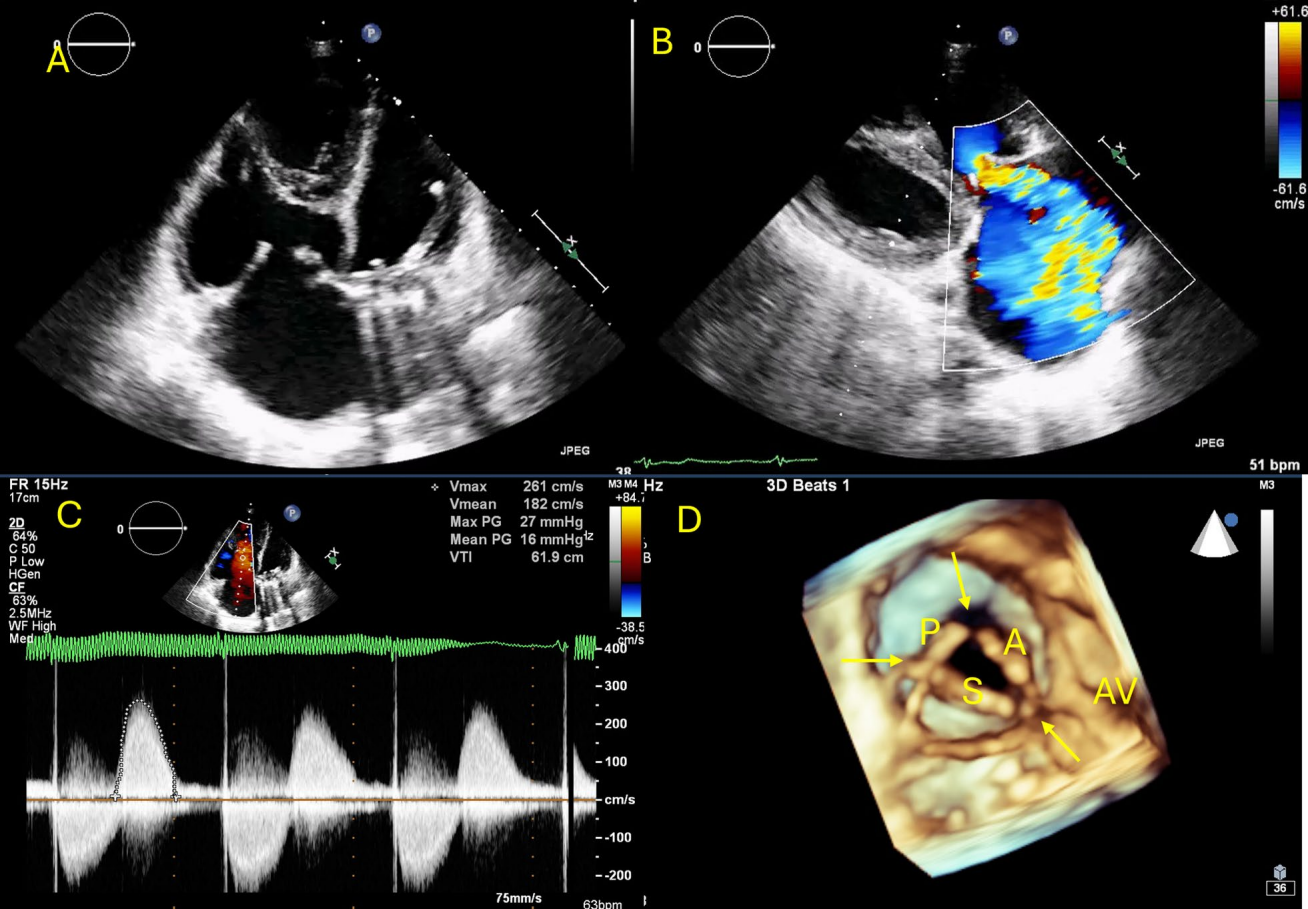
2D/3D imaging of the tricuspid valve in carcinoid heart. A RV-focused apical view showed thickened leaflets with reduced excursion and lack of coaptation. B Massive tricuspid regurgitation on color Doppler. C Mean transvalvular gradient of 16 mmHg. D En face visualization of the TV from the RV perspective showing a unique commissural soaring pattern
